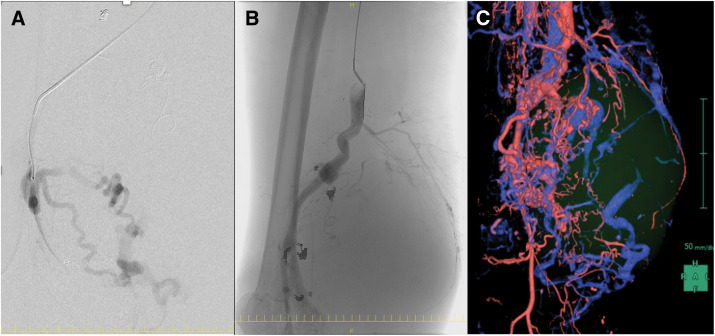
Coil embolization findings. A total of 29 coils were used to embolize abnormal vessels extending from the SFA to the hematoma over 8 h (A). Post-embolization DSA showed a reduction in the AVM extending the hematoma (B). Unfortunately, post-embolization CTA revealed the persistence of numerous arteriovenous fistulas (C). AVM, arteriovenous malformation; SFA, superficial femoral artery
